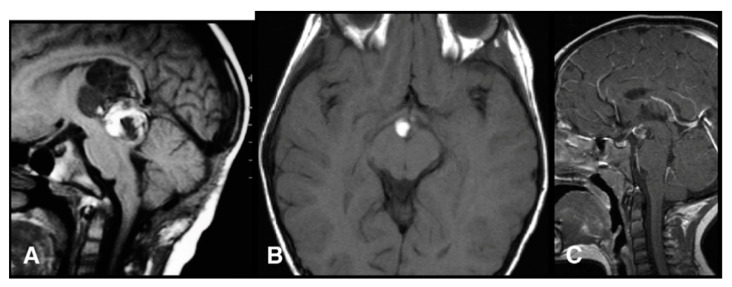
Pineal teratoma MRI. Sagittal T1 image (A), axial T1 (B) and sagittal contrast-enhanced T1 (C) images. Reprinted with permission from Peterson et al. (2012) [54]. Elsevier—2021 (License Number: 5034851378505).
